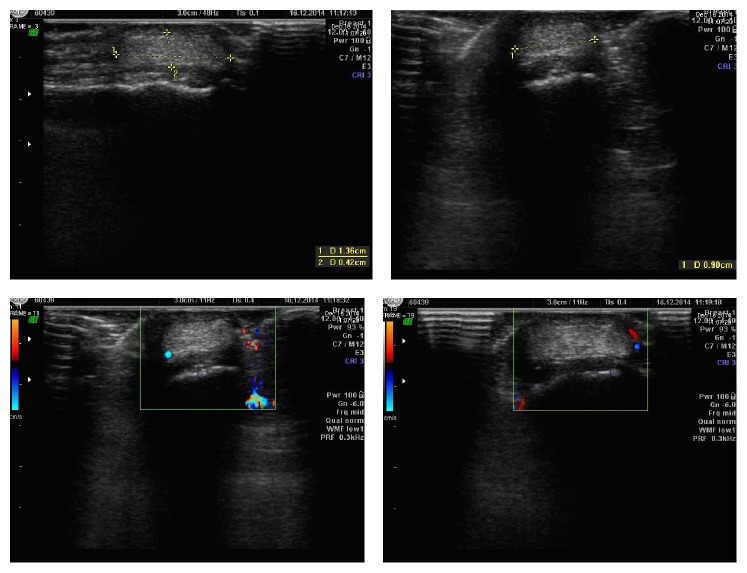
Ultrasound of the ulnar aspect of the thumb showing a well defined mildly hyperechoic lesion measuring 1.36*∗*0.42*∗*0.9 cm abutting the ulnar collateral ligament, which is otherwise intact. No internal flow was seen on Doppler.
